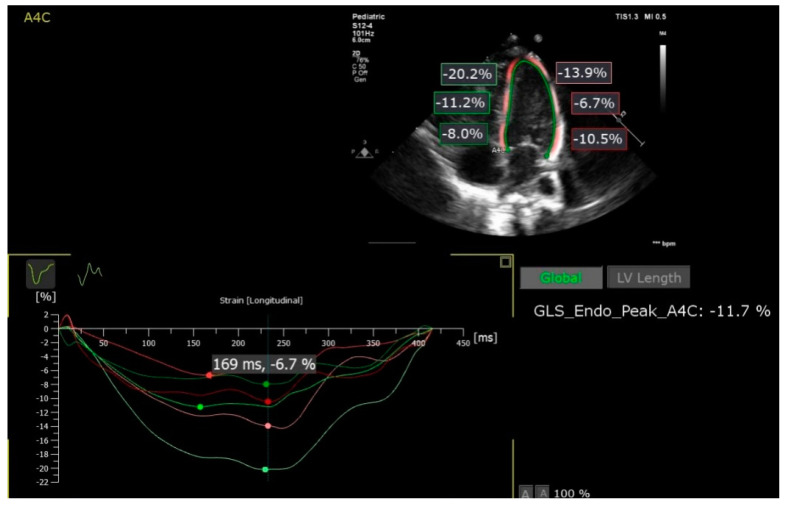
Left ventricle speckle tracking analysis; GLS_Endo_Peak_A4C (denoted by LV pGLS in our study)—peak global longitudinal strain of the left ventricle from apical 4-chamber view (autostrain LV, PhlipsQlab 13).
